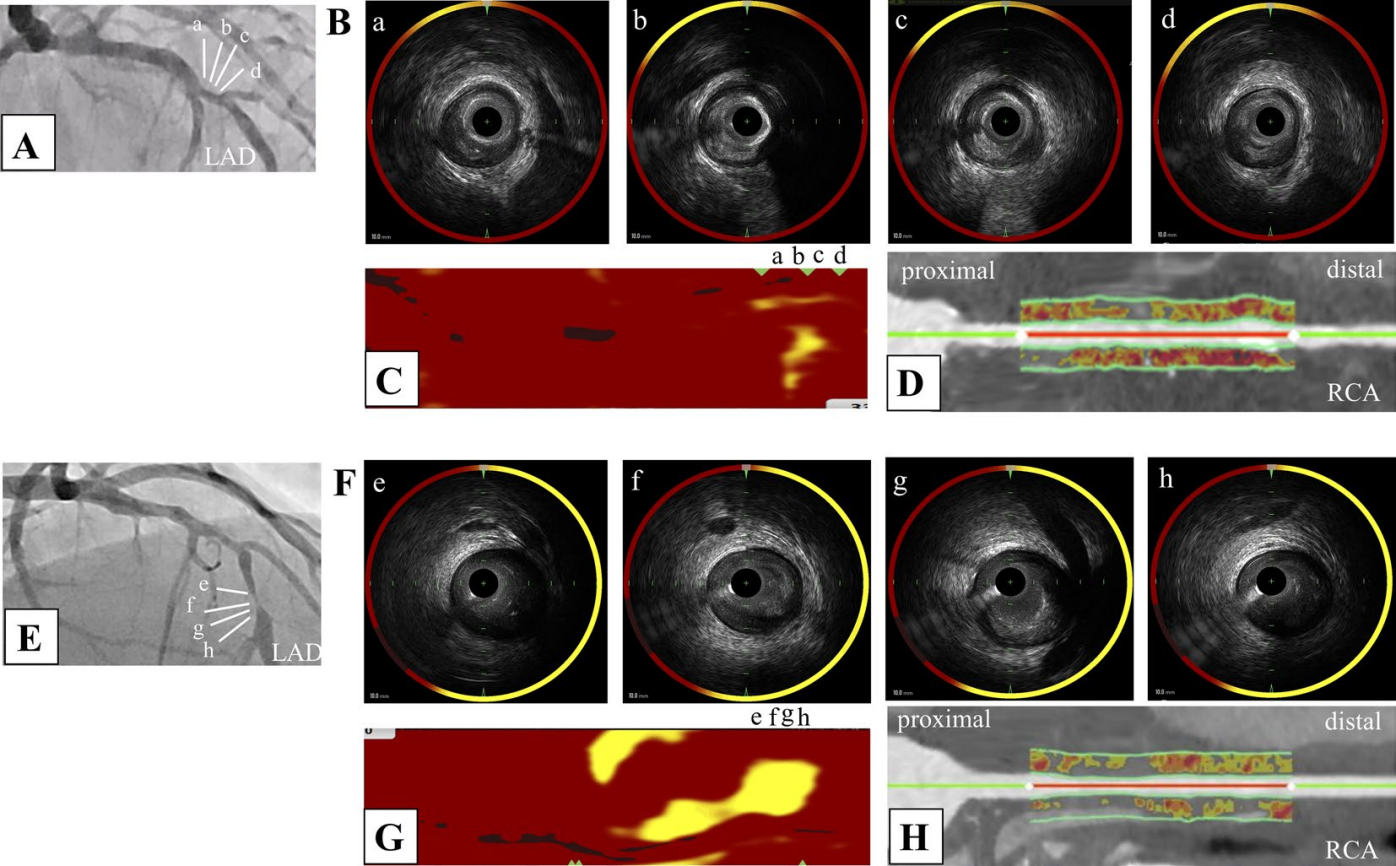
Representative cases with or without high vascular inflammation. A 77 years old male with stable coronary artery disease. Coronary angiography demonstrated that moderate stenosis in his middle left descending artery (LAD). B Grey-scale intravascular ultrasound (IVUS) showed calcified plaque with no attenuation in the culprit lesion. C Near-infrared spectroscopy chemogram revealed that there was less lipid accumulation in the culprit lesion (maxLCBI_4mm_ = 292). D In coronary computed tomography angiography (CCTA) analysis, his pericoronary adipose tissue attenuation at proximal RCA was low (PCAT_RCA_ attenuation = − 98.1 HU). E 85 years old male with stable coronary artery disease. Coronary angiography demonstrated that severe stenosis in his middle LAD. F Grey-scale IVUS showed low echoic plaque with attenuation in the culprit lesion. G NIRS chemogram revealed that there was large lipid burden in the culprit lesion (maxLCBI_4mm_ = 465). H In CCTA analysis, he had high coronary artery inflammation (PCAT_RCA_ attenuation = − 72.7 HU)
